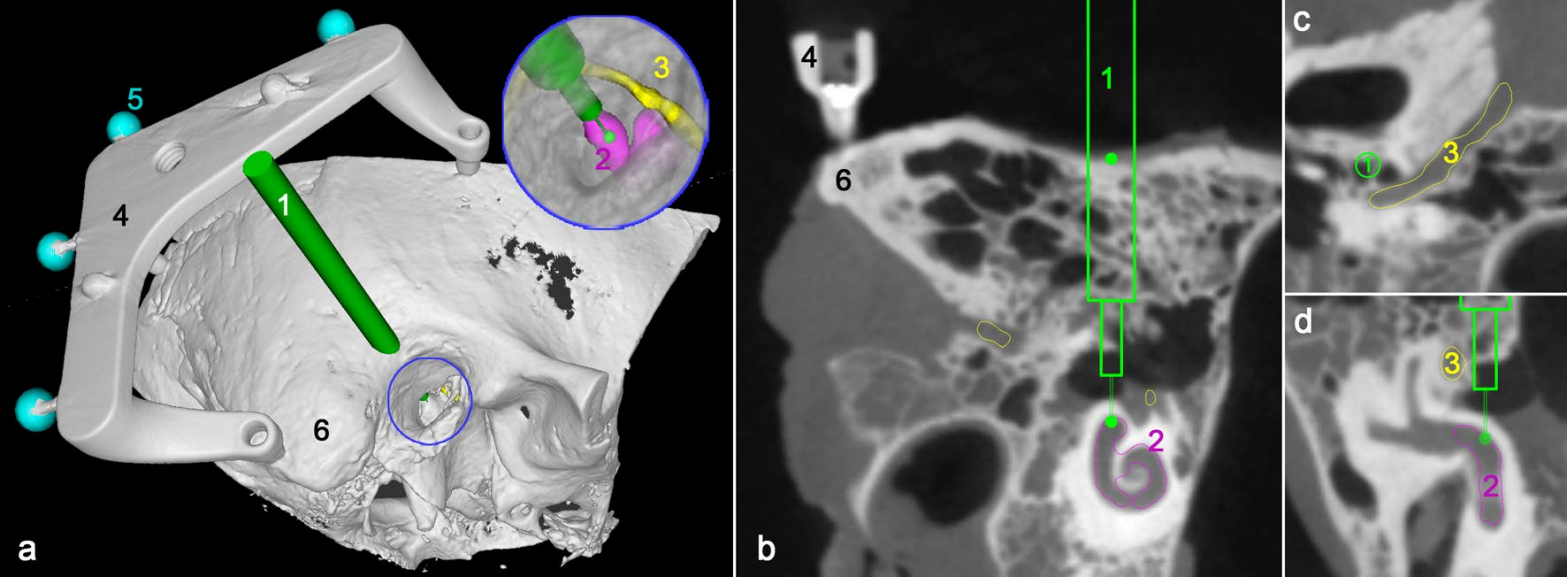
Screenshots of the planning. a 3D view of the planned trajectory (1) targeting the cochlea (2) while passing the facial nerve (3). The Trifix (4) with its registration markers (5) is fixed to the temporal bone (6). b Sectional view of the CBCT image in the plane of the trajectory, c Path of flight view perpendicular to the planned trajectory showing its spatial relation to the facial nerve. d Trajectory with planned safety margin to the facial nerve
